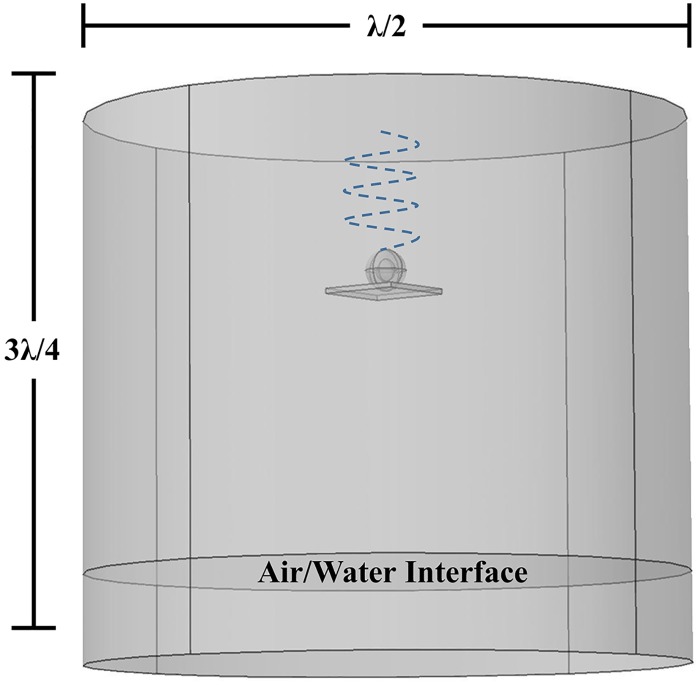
Model geometry. Cell attached to a planar surface (polystyrene to mimic the properties of a 6-welled plate) immersed in fluid (growth media). The transducer (ultrasound source) is positioned at the top. The cell position changes so that it always lies at an antinode or node.
